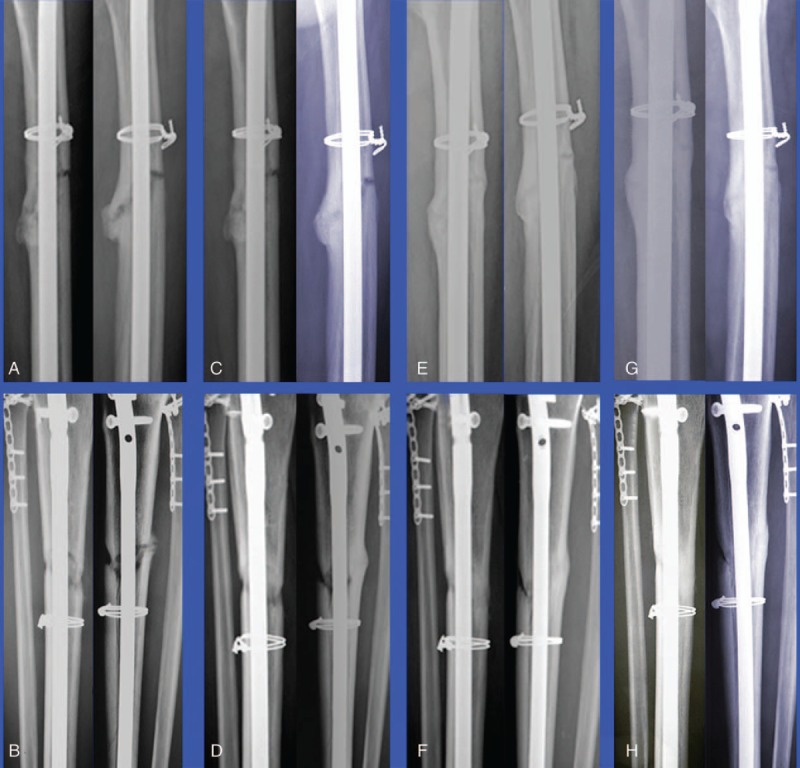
Serial anteroposterior and lateral plain radiographs of left tibial and right femoral fracture nonunion during treatment. (A and B) Nonunion before treatment. (C and D) Nonunion after a 4-month course of teriparatide. (E and F) Nonunion after an 8-month course of teriparatide. (G and H) Healing of nonunion at 4 months after discontinuation of teriparatide.
